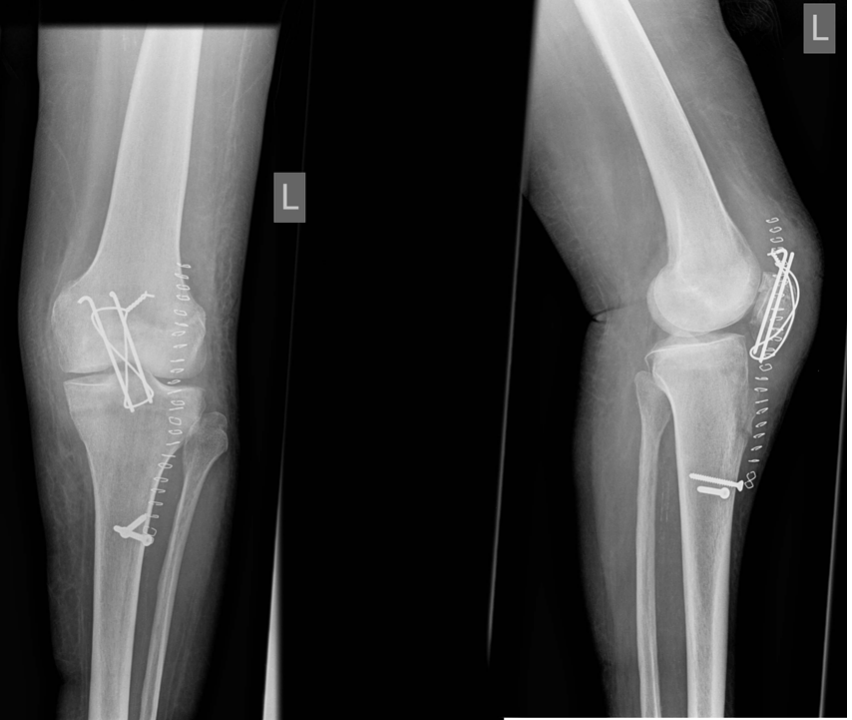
Postoperative anteroposterior and lateral radiographs of left knee after refixation of avulsion fracture of the tibial tuberosity.
